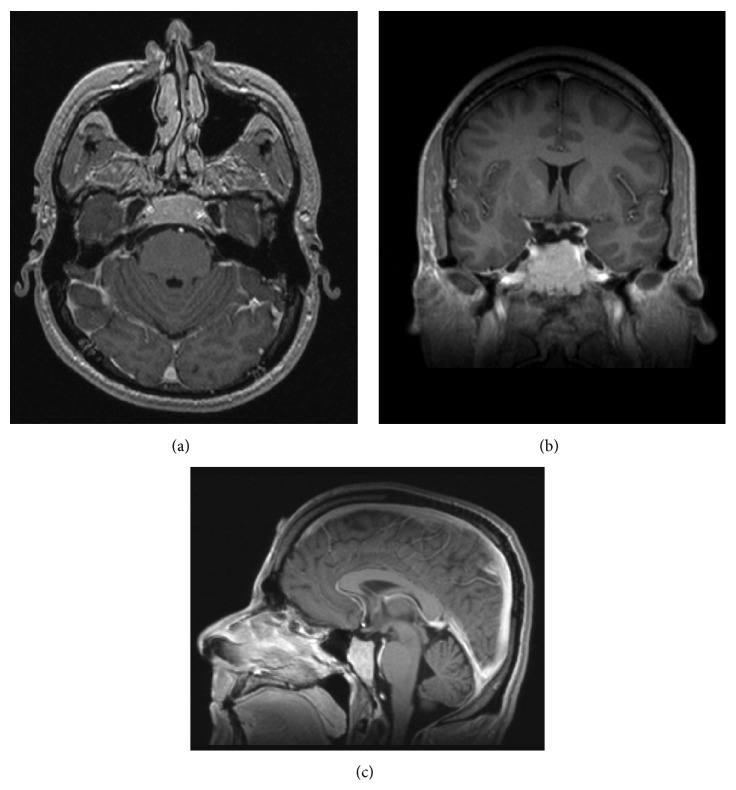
Preoperative magnetic resonance imaging, with contrast, axial (a), coronal (b), and sagittal (c) sections, demonstrating contrast enhancing, clival tumor.
